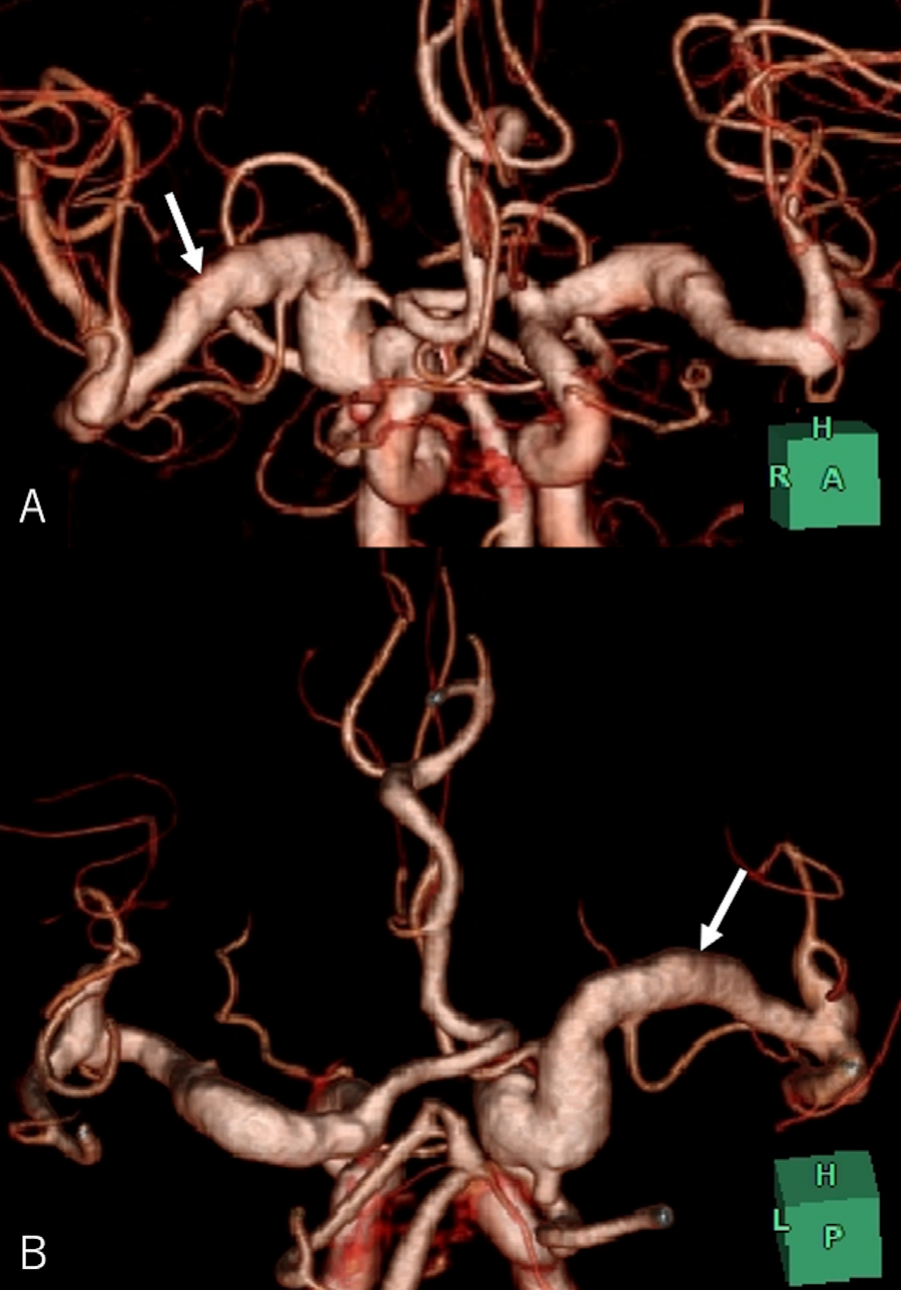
Three-dimensional computed tomography angiography, anteroposterior (*A*) and posteroanterior (*B*) views, performed 3 months after injury, showing walls of the dolichoectatic right middle cerebral artery without de novo abnormalities (*arrow*).
